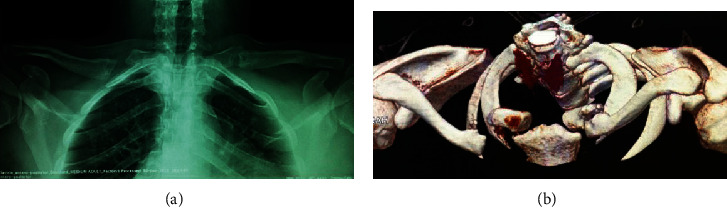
After two years follow up, (a) Anteroposterior radiograph of the clavicles revealing bilateral pseudoarthrosis. Incidence performed with a 40° head rays (serendipity incidence). (b) Computed tomography with three-dimensional reconstruction of the clavicles characterizing the pseudoarthrosis.
